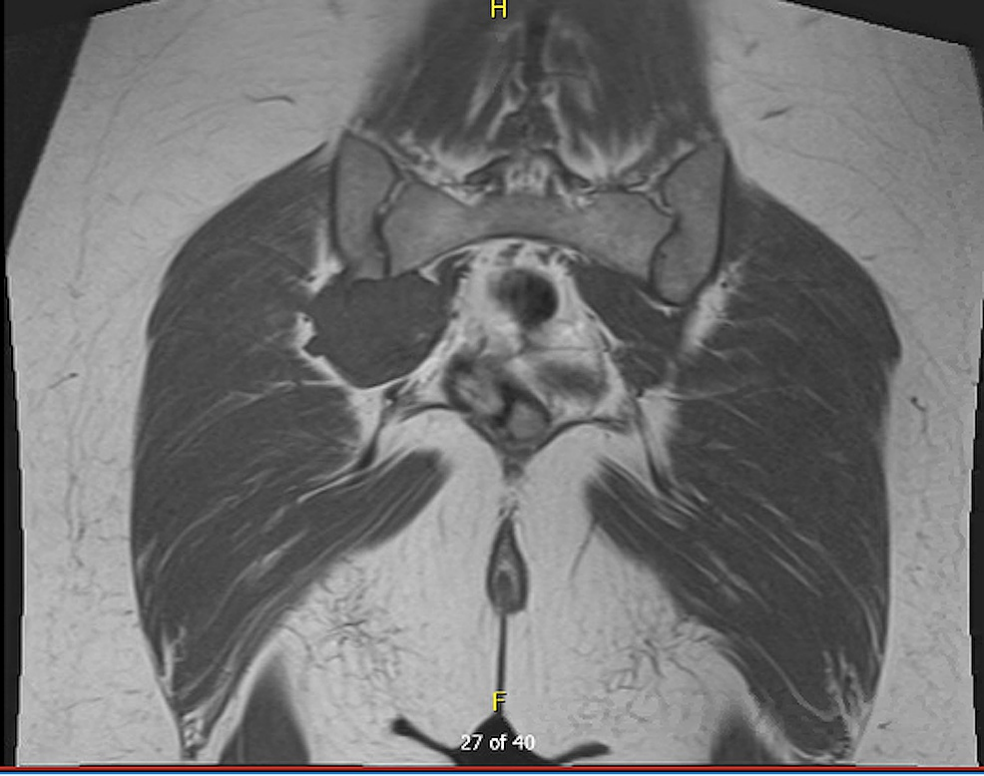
Coronal view of T1. MRI showing 6 cm x 5.6 cm x 4.5 cm mass along the right ilium extending into the ipsilateral sacroiliac joint. MRI showing 6 cm x 5.6 cm x 4.5 cm mass along the right ilium extending into the ipsilateral sacroiliac joint.
